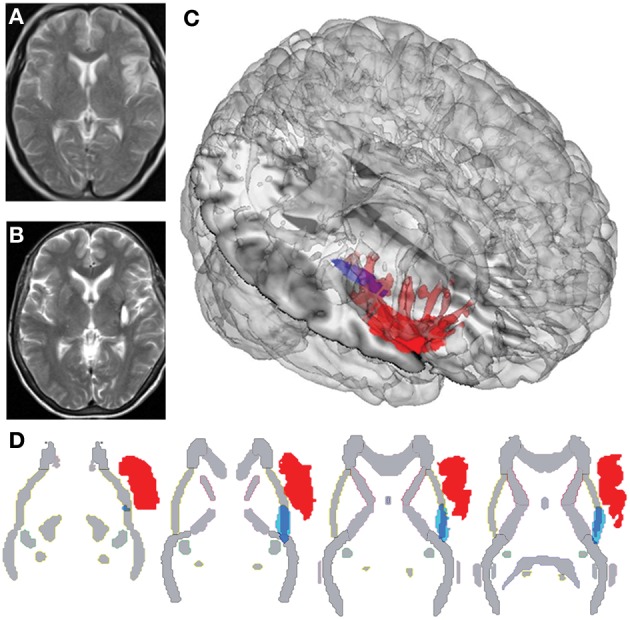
Different plots of IL and SL brain damage localization. (A) Structural MRI of IL, with sequence T2 showing the right insular cortex lesion. (B) Structural MRI of SL, with sequence T2 showing damage to the right posterior putamen, capsula extrema, *claustrum*. (C) Three-dimensional rendering of lesion-traced MNI-normalized brain lesions of IL and SL plotted onto a standard T1 glass brain with the Mango software. (D) Overlap of lesion-traced MNI-normalized brain lesions of IL and SL and the JHU-Atlas of white matter, showing differential affectation of external capsule in both lesions.
